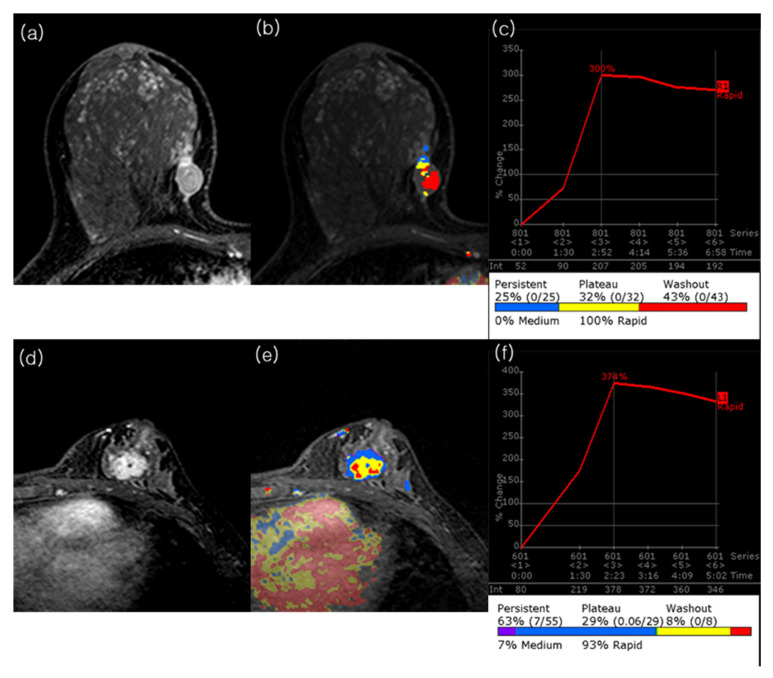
Representative preoperative MRI cases of TNBC with contrasting angiovolume and outcomes. (a–c) A 40-year-old woman without recurrence during follow-up. Peak dynamic image shows an irregular, rim enhancing 1.6 × 1 × 1.6 cm (calculated tumor volume = 1.34 cm^3^) mass in the right breast. The CAD color map demonstrates prominent intratumoral areas below the threshold, corresponding to an angiovolume of 1.1 cm^3^. The automatically generated portfolio indicates a peak enhancement of 300%. The pathologic tumor size was 2.0 cm, with no axillary lymph node metastasis. (d–f) A 39-year-old woman with ipsilateral breast cancer and axillary nodal recurrence at 12.7 months after surgery, followed by cervical and mediastinal lymph node metastases at 52.7 months. Peak dynamic image shows an irregular, heterogeneously enhancing 2.4 × 1.8 × 2.4 cm (calculated tumor volume = 5.43 cm^3^) mass in the left breast. The CAD color map demonstrates nearly complete tumor enhancement, corresponding to an angiovolume of 5.4 cm^3^. The portfolio indicates a peak enhancement of 374%. The initial pathologic tumor size was 2.0 cm, with axillary lymph node metastasis.
